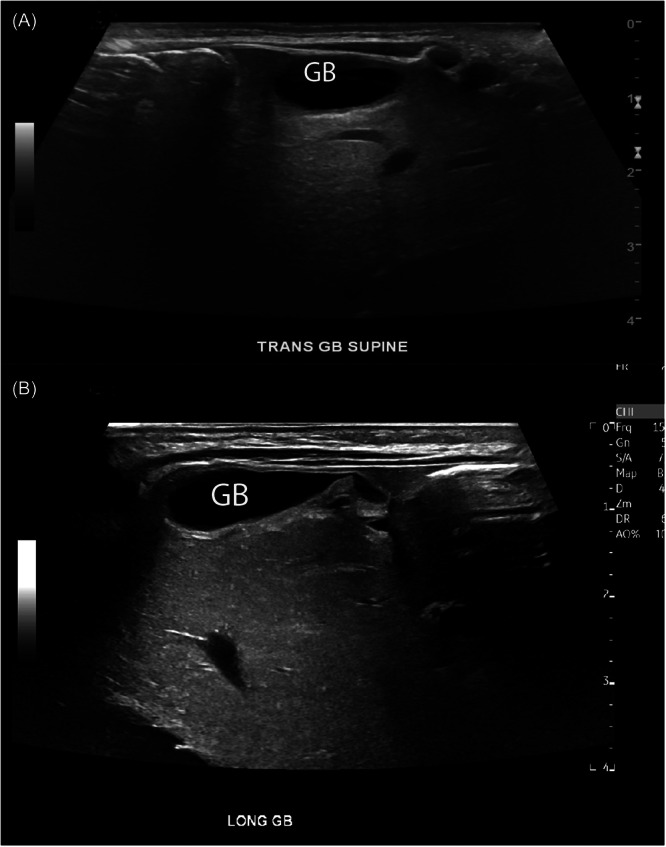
Abdominal ultrasound performed at age 1 day shows abnormal position of GB. Transverse (A) and longitudinal (B) show GB positioning at the anterior surface of the left hepatic lobe, rather than as expected under the surface of the right hepatic lobe. GB, gallbladder.
